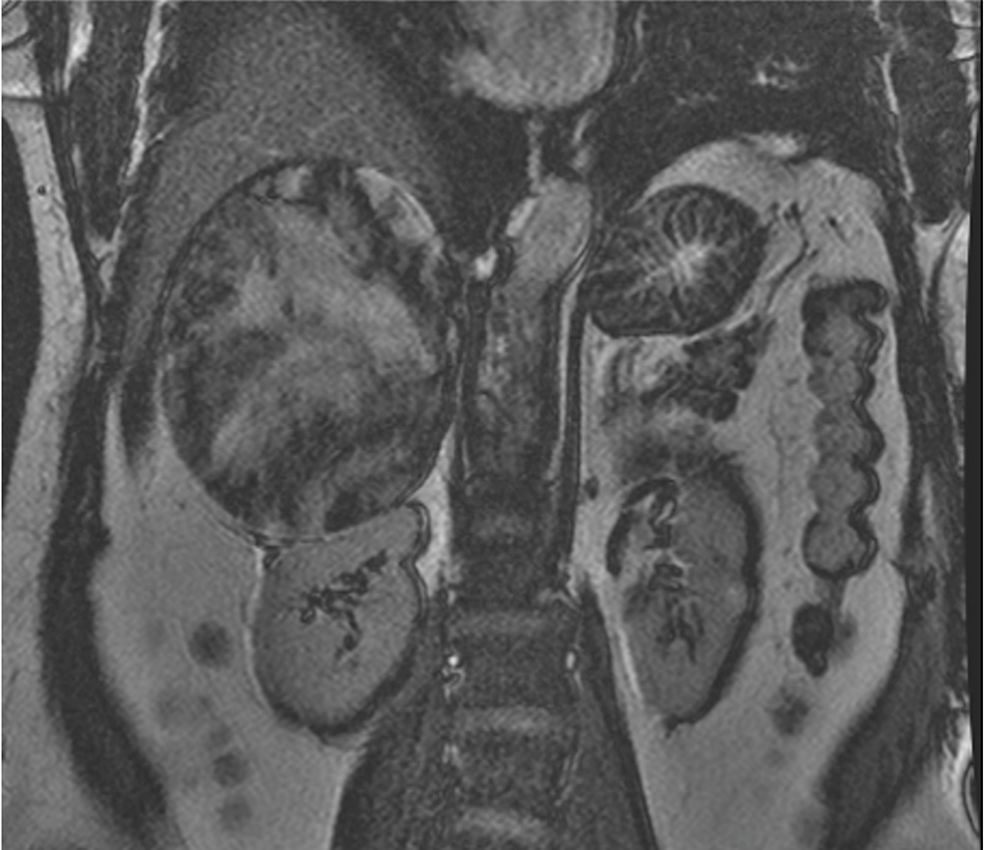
MRI 2-d Fast Imaging Employing Steady-State Acquisition Non-Fat Saturated - Coronal View Image demonstrates a large right adrenal gland lesion with mass effect on right kidney.
